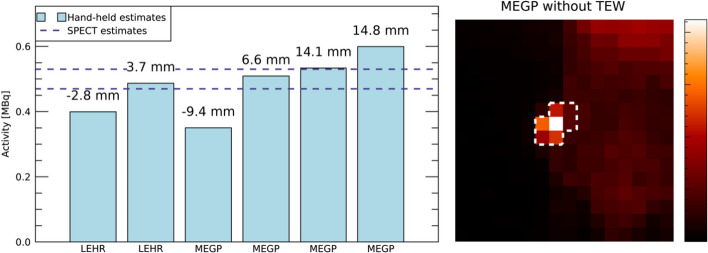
Estimates of tumour activity from the pre-clinical measurements (left) with data from the hand-held camera (bars) and estimates from the SPECT/CT by two independent operators (horizontal dashed lines). The depths estimated from the hand-held measurements are indicated above each corresponding bar. The right panel shows an image acquired with the hand-held camera, with the 113 keV window and MEGP collimator. The ROI used for activity quantification is indicated by a white dashed line
